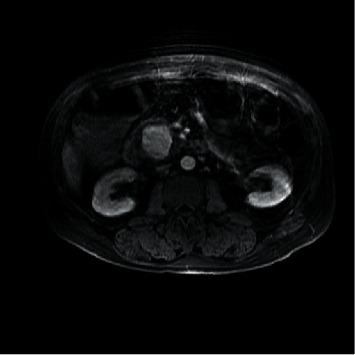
Magnetic resonance imaging without measurement of pseudoaneurysm.
